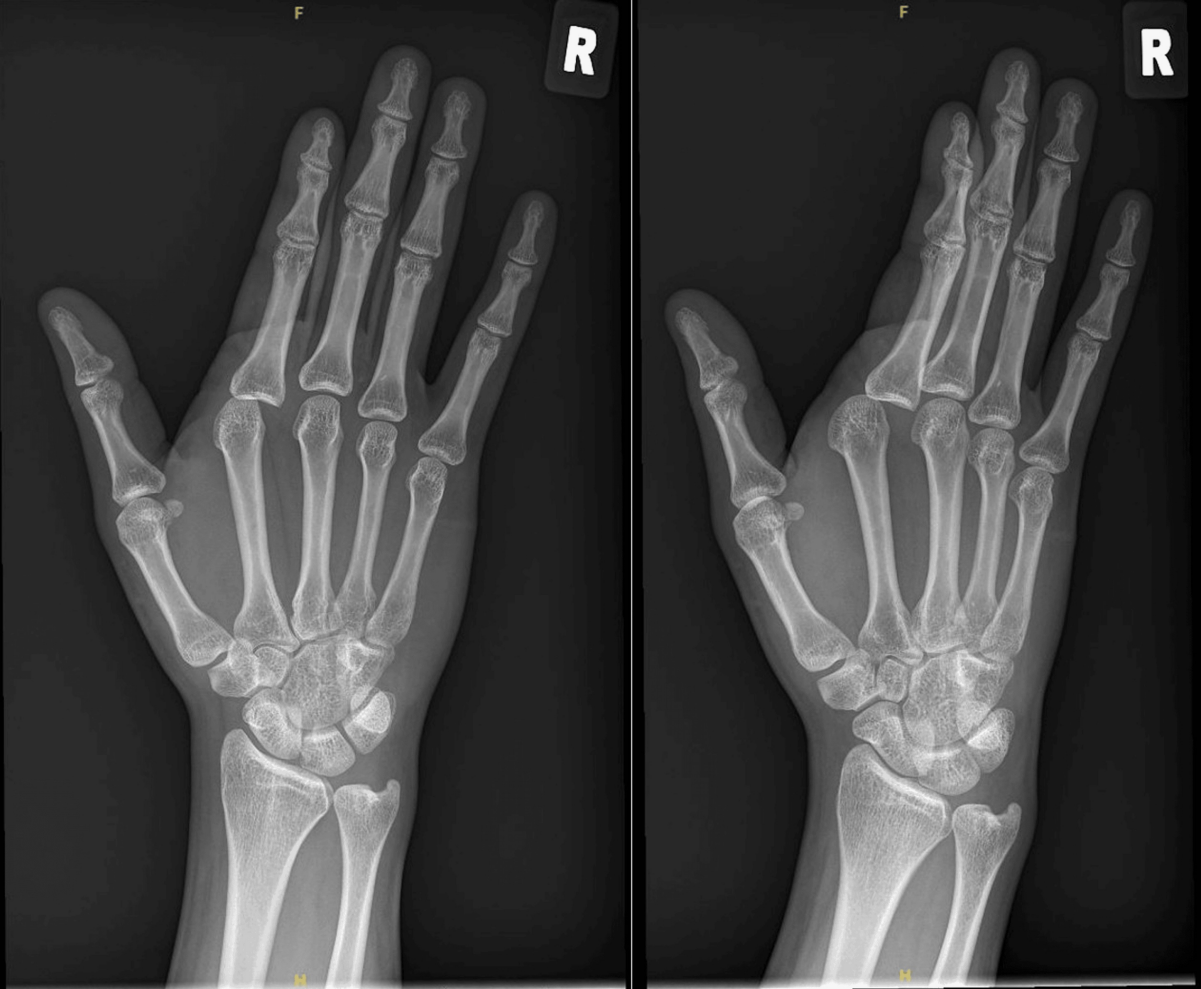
Plain radiographs of the patient’s right hand showing right index finger MCPJ dislocation MCPJ: metacarpophalangeal joint
